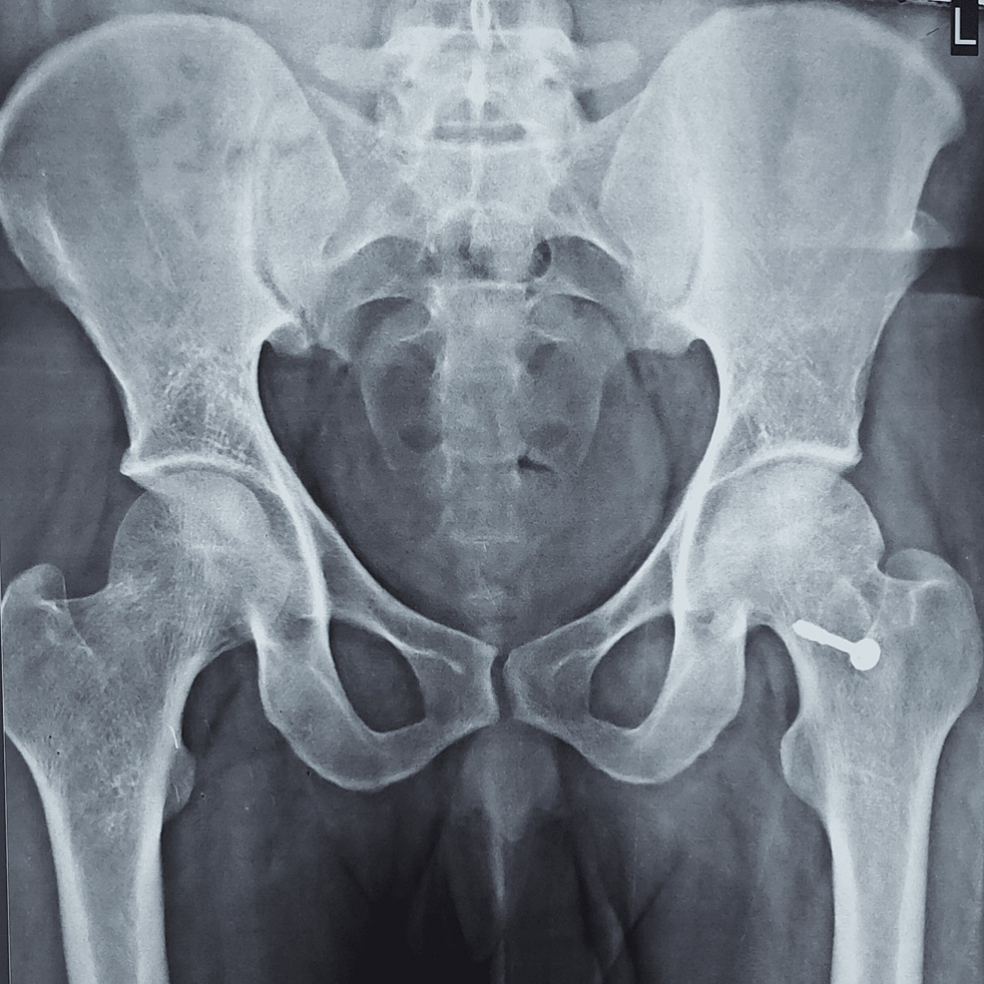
Postoperative radiograph at 10 years of final follow-up
